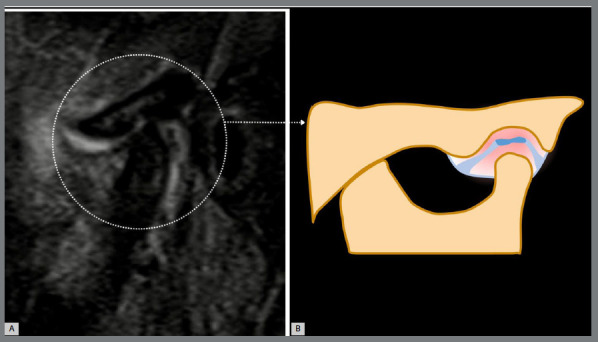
Inflammatory phenotype: a bright signal inside the joint spaces that has a convex configuration in the anterior and/or posterior recesses, and an irregularly condylar head and/or articular fossa contour. A) Referential temporomandibular joint MRI sagittal T2-weighted image. B) Temporomandibular joint scheme. Adapted from ROAMES - Rapid OsteoArthritis MRI Eligibility Score (Source: Roemer et al.^8^, 2020).
